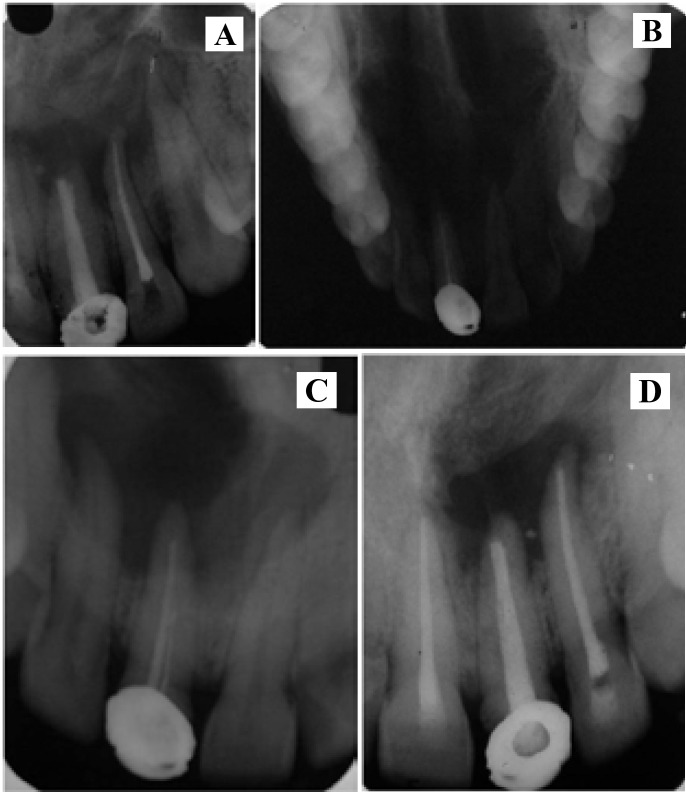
A.- Maxillary occlusal view. B. - Pre-operative Intraoral periapical radiograph. C. - months post operative radiograph. D. - 6 months post operative radiograph.
